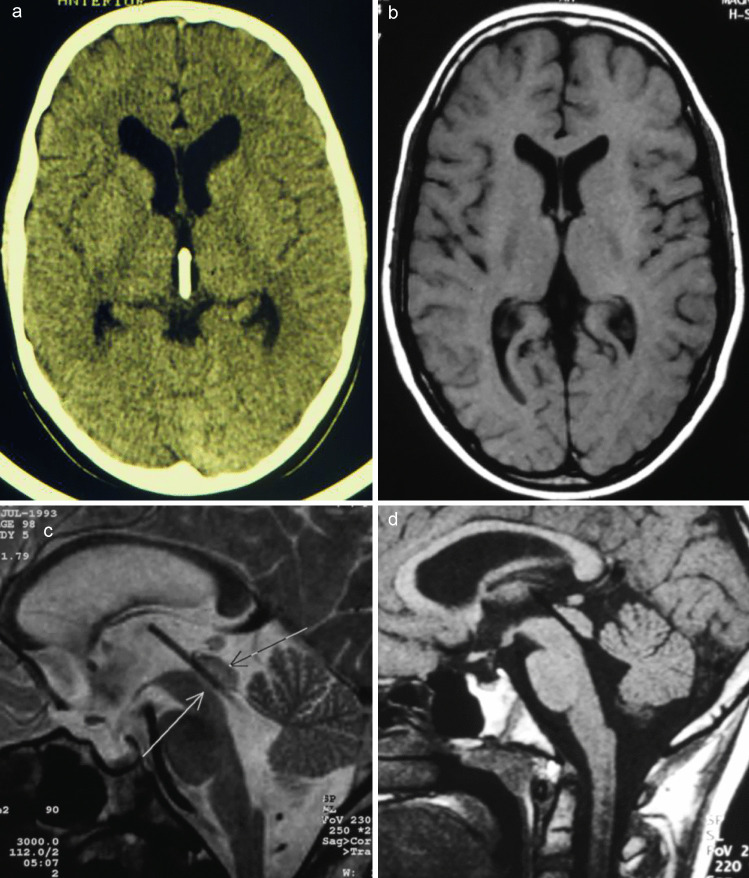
Postoperative obtained axial computed tomogram a axial and sagittal MR images b, c, d showing the optimal positioning of the transaqueductal stents. Additionally, the obvious reduction of the lateral and the third ventricle size indicated the functionality of the stent
